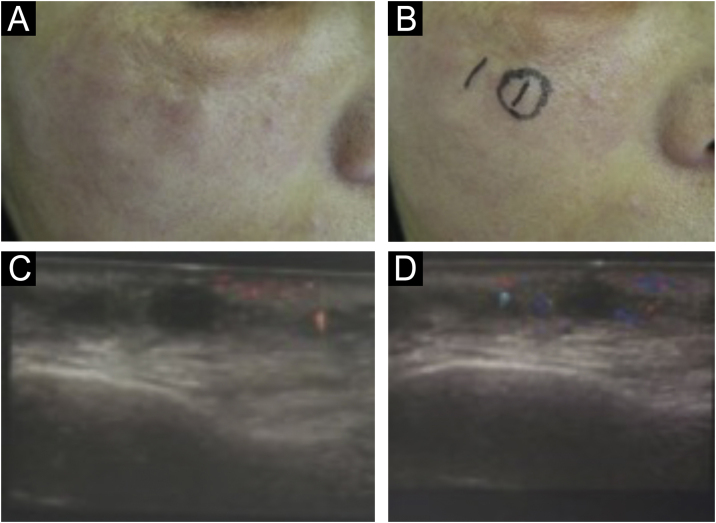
(A‒D) Inflammatory reactivation in sites treated with biomaterial after distant infection, in this case, tonsillitis. (C and D) Ultrasound appearance: increased vascularization around the biomaterial.
